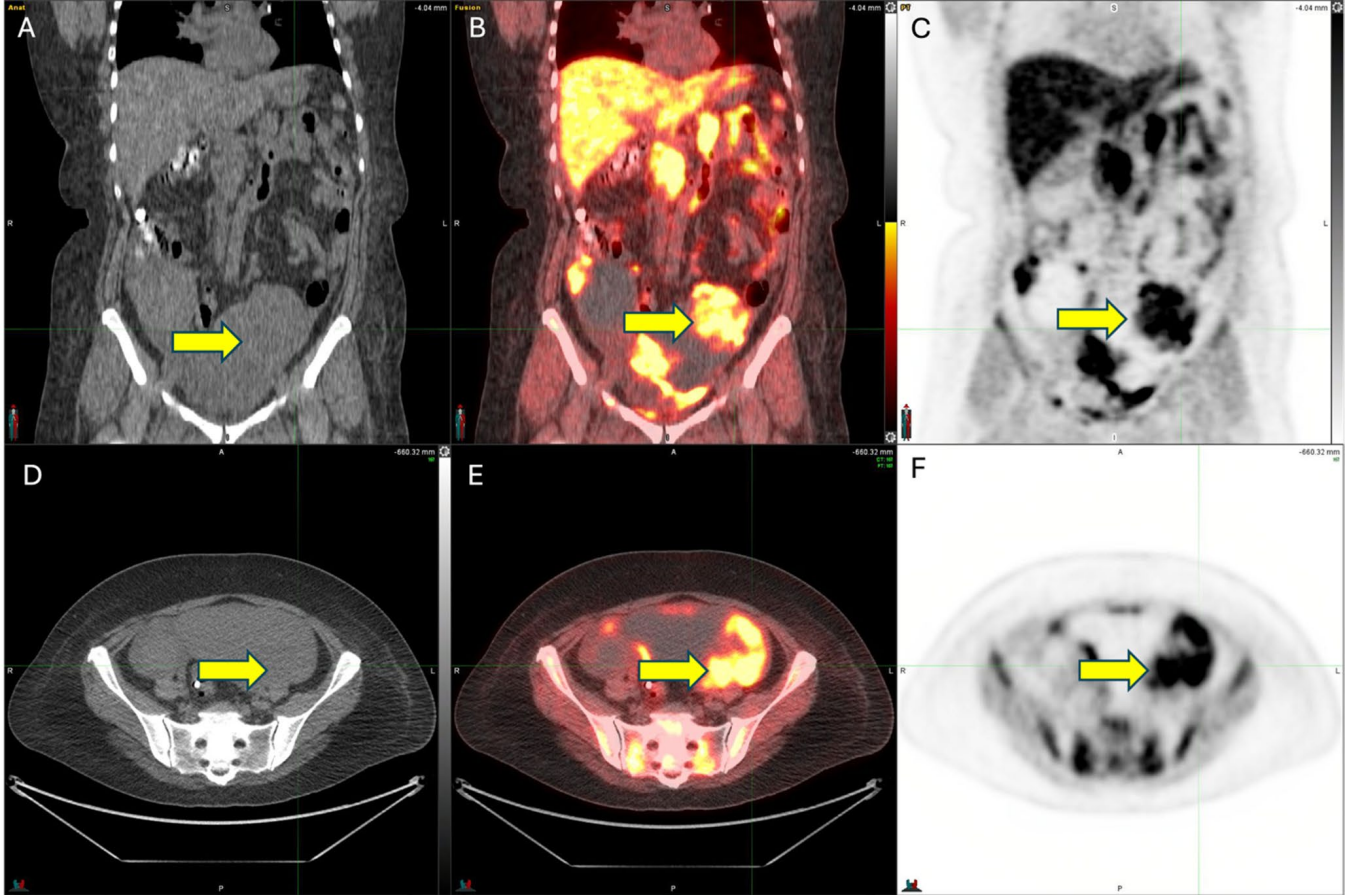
Images are from a 52-year-old woman with a germline *BRCA1* mutation high-grade serous ovarian cancer showing avid ^18^F-FTT uptake in the left ovary (outlined in purple, yellow arrow) on coronal Non-Contrast CT (A), [^18^F]-FTT PET/CT (B) and [^18^F]-FTT PET (C) and axial Non-Contrast CT (D), [^18^F]-FTT PET/CT (E) and [^18^F]-FTT PET (F) with SUVmax = 9 (green circle refers to point of maximum activity), tumor to muscle ratio (T/M) = 4.9
